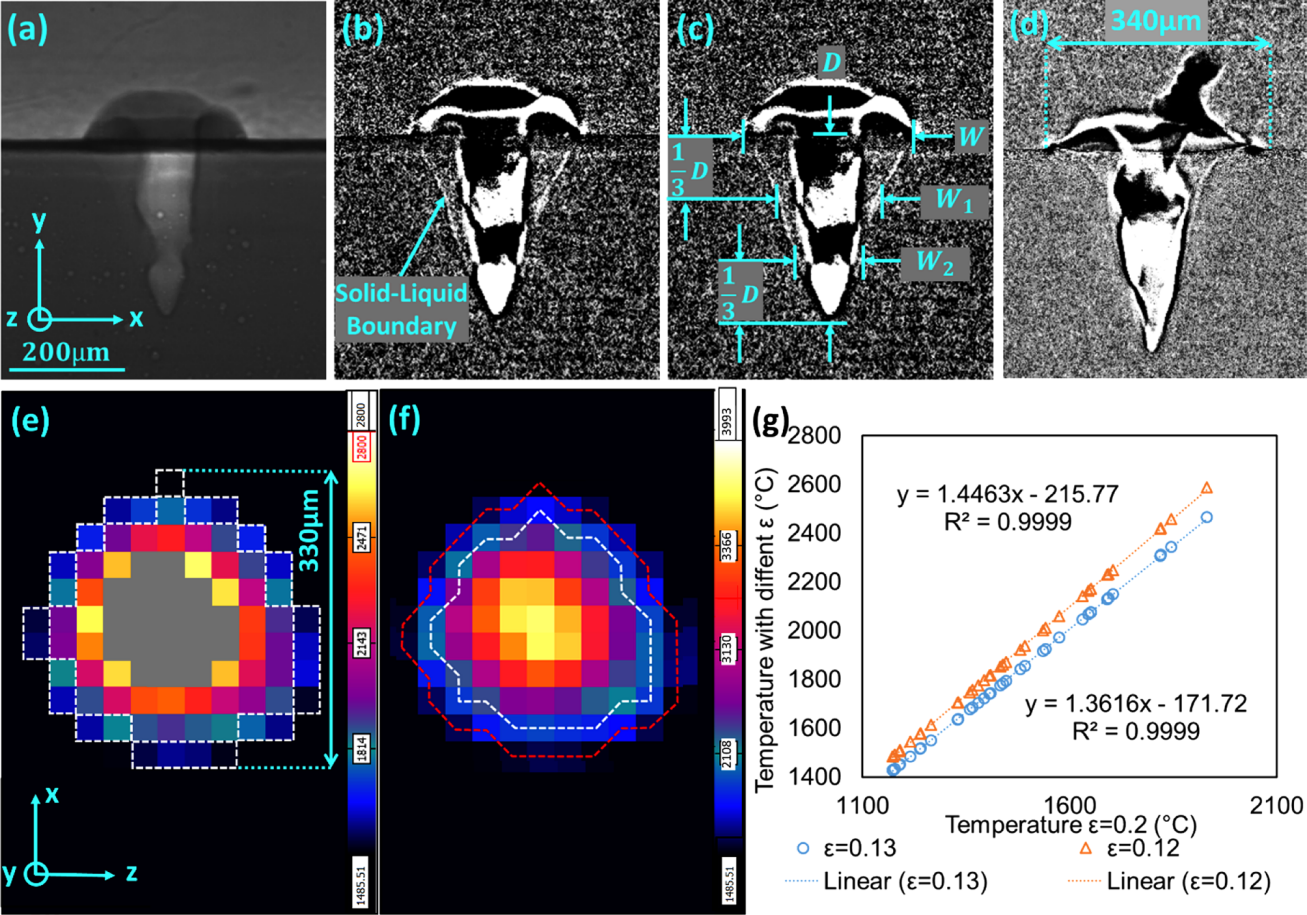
The (a) un-processed and (b) processed high-speed X-ray imaging of a 410 SS melt pool. The backgrounds have all been removed in the processed one, and the boundary of the melt pool becomes clear. (c) The definition of the melt pool dimension terms. (d) The processed synchronized high-speed X-ray image (Trial #12) at t = 0.4 ms (the first 1.1 ms is the laser delay time). (e) The high-speed IR image (*ε* = 0.12) of the melt pool at the same timestamp. The white dash region represents the pixels used for average solid–liquid boundary temperature calculation, and the grey-color region represents invalid readings due to temperature exceeding manufacture calibration temperature. (f) The corrected high-speed IR image (*ε* = 0.12) of the melt pool at the same timestamp. Notice the missing value problem has been solved. (g) The temperature conversion between different emissivity values. (Fig. 2d–g is discussed in "Thermal camera calibration, missing data interpretation, and thermal terms calculation methods" Section, and the temperatures in Fig. 2e and f are all in °C).
